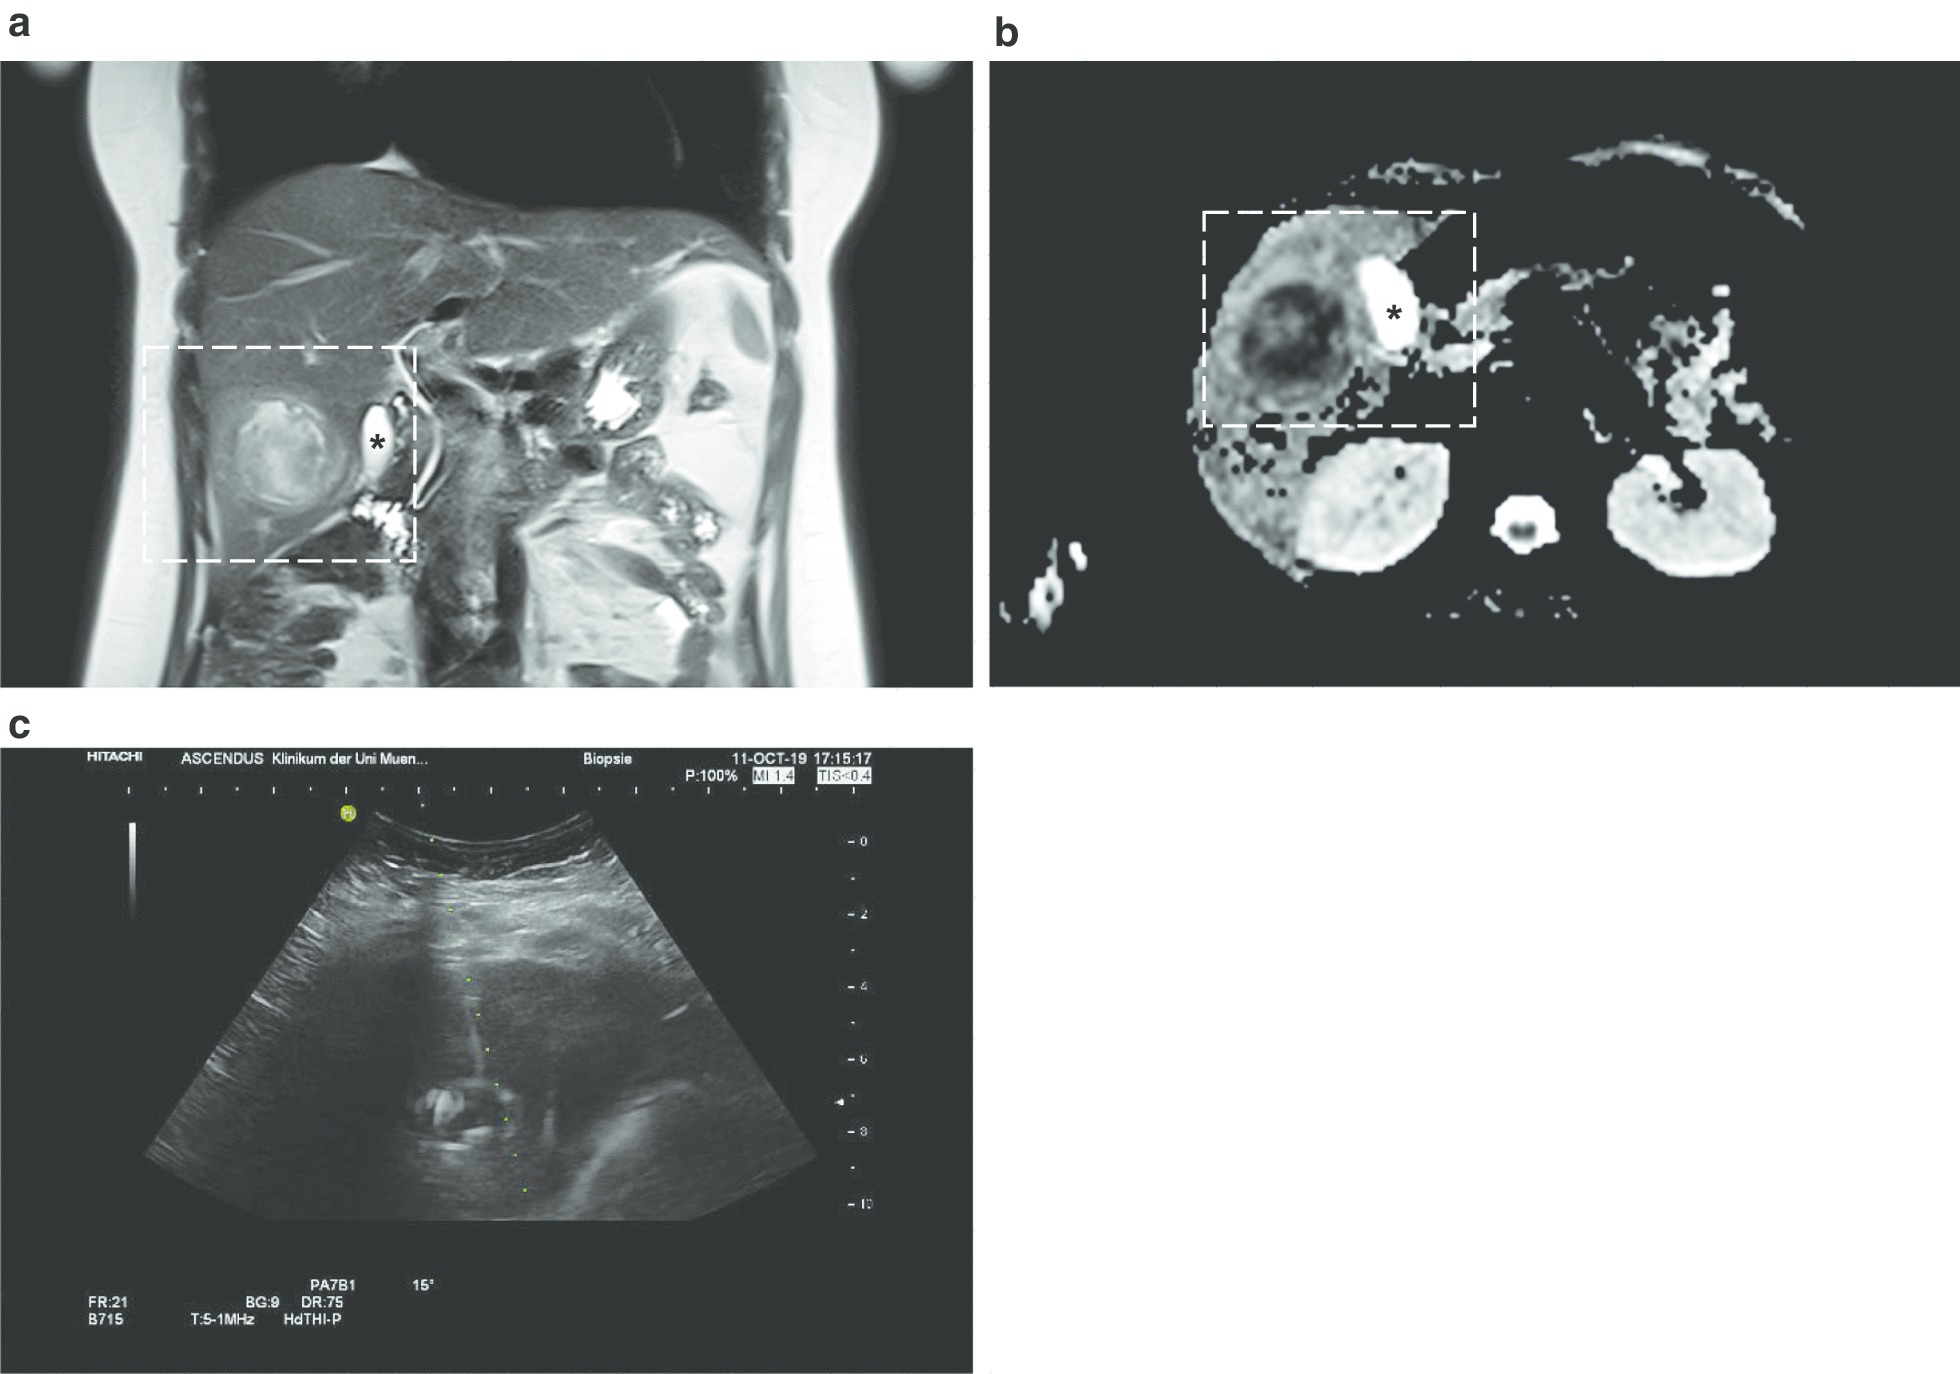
a Coronary MRI image of the liver without contrast reveals a T1-hypointense hepatic lesion, adjacent to the gall blader (indicated with *). b Axial MRI sequences showing diffusion restriction to the center of the lesion (* = gall bladder). c 2D sonography after successful transhepatic puncture and drainage. The green dots depict the direction of puncture, the hyperechogenic reflexes within the lesion are caused by the drainage
